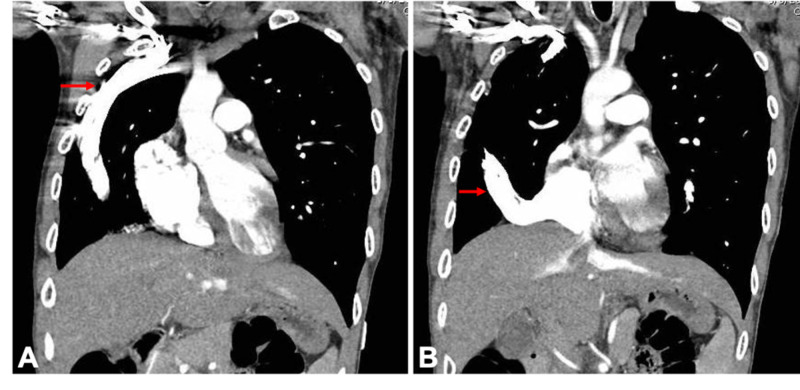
CT angiogram of the chest (coronal sections). (A) Superior portion of the large anomalous superior vena cava draining the right jugular, subclavian and innominate vein that extends caudally in the anterior pleural space. (B) Inferior portion of the same anomalous superior vena cava communicating with the inferior vena cava at the junction of the right atrium.
